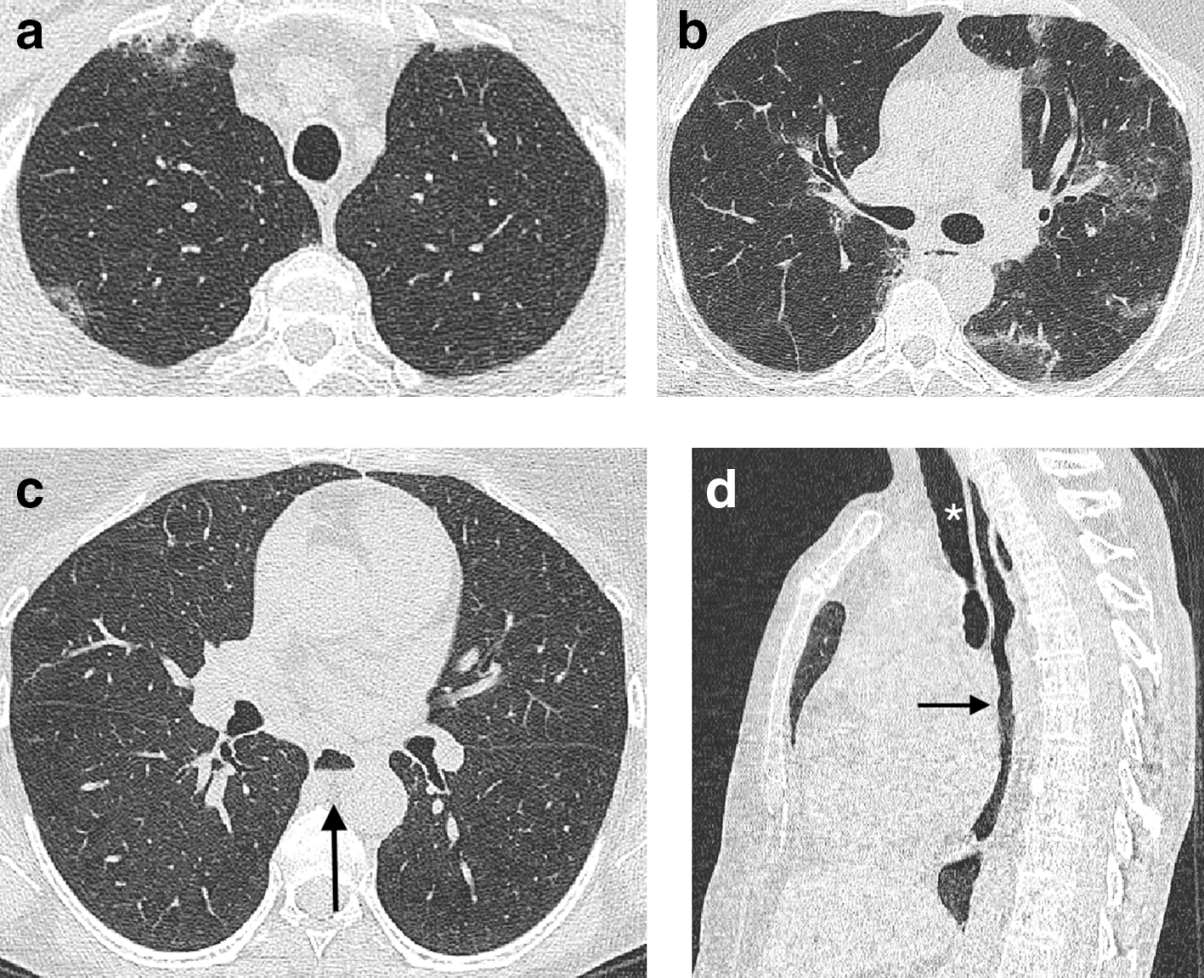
Organizing pneumonia in the upper (a) and middle (b) zones in a patient with polymyositis. The organizing pneumonia is predominantly located peripherally in the lung and is characterized by arcs of dense fibrosis surrounded by ground glass density in keeping with the reverse halo or Atoll sign. Organising pneumonia can demonstrate a wide variety of appearances on CT. A dilated patulous oesophagus (arrows) on axial (c) and sagittal (d) CT imaging in a patient with scleroderma. An air-fluid level is visible in the oesophagus on axial imaging; the trachea is demarcated with an Asterix.
